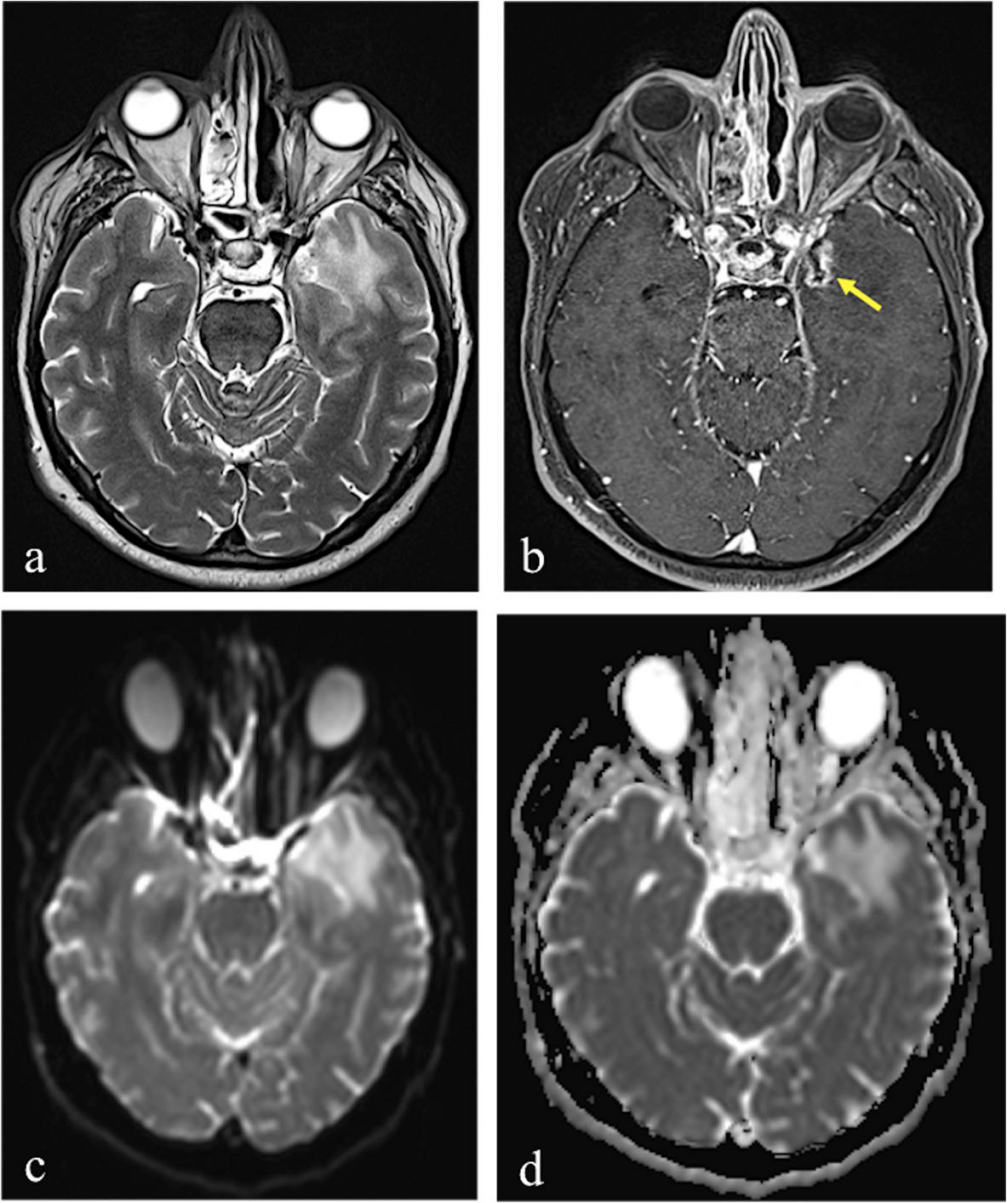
MRI performed 20 months after 68.8 Gy carbon ion therapy in a patient who previously underwent surgical resection for sinonasal adenoid cystic carcinoma. Axial T2-weighted image (a) shows hyperintensy involving subcortical white matter and gray matter in left polar and mesial temporal regions. Axial post-contrast T1-weighted image (b) reveals the presence of a well-defined contrast-enhancing lesion with central necrotic core (yellow arrow), as in grade 2 injury of LENT-SOMA scoring system. DWI shows no diffusion restriction within the enhancing area, with an hyperintense signal in both *DWI*-*b1000* (c) and ADC map (d), because of “T2-shine through” effect
